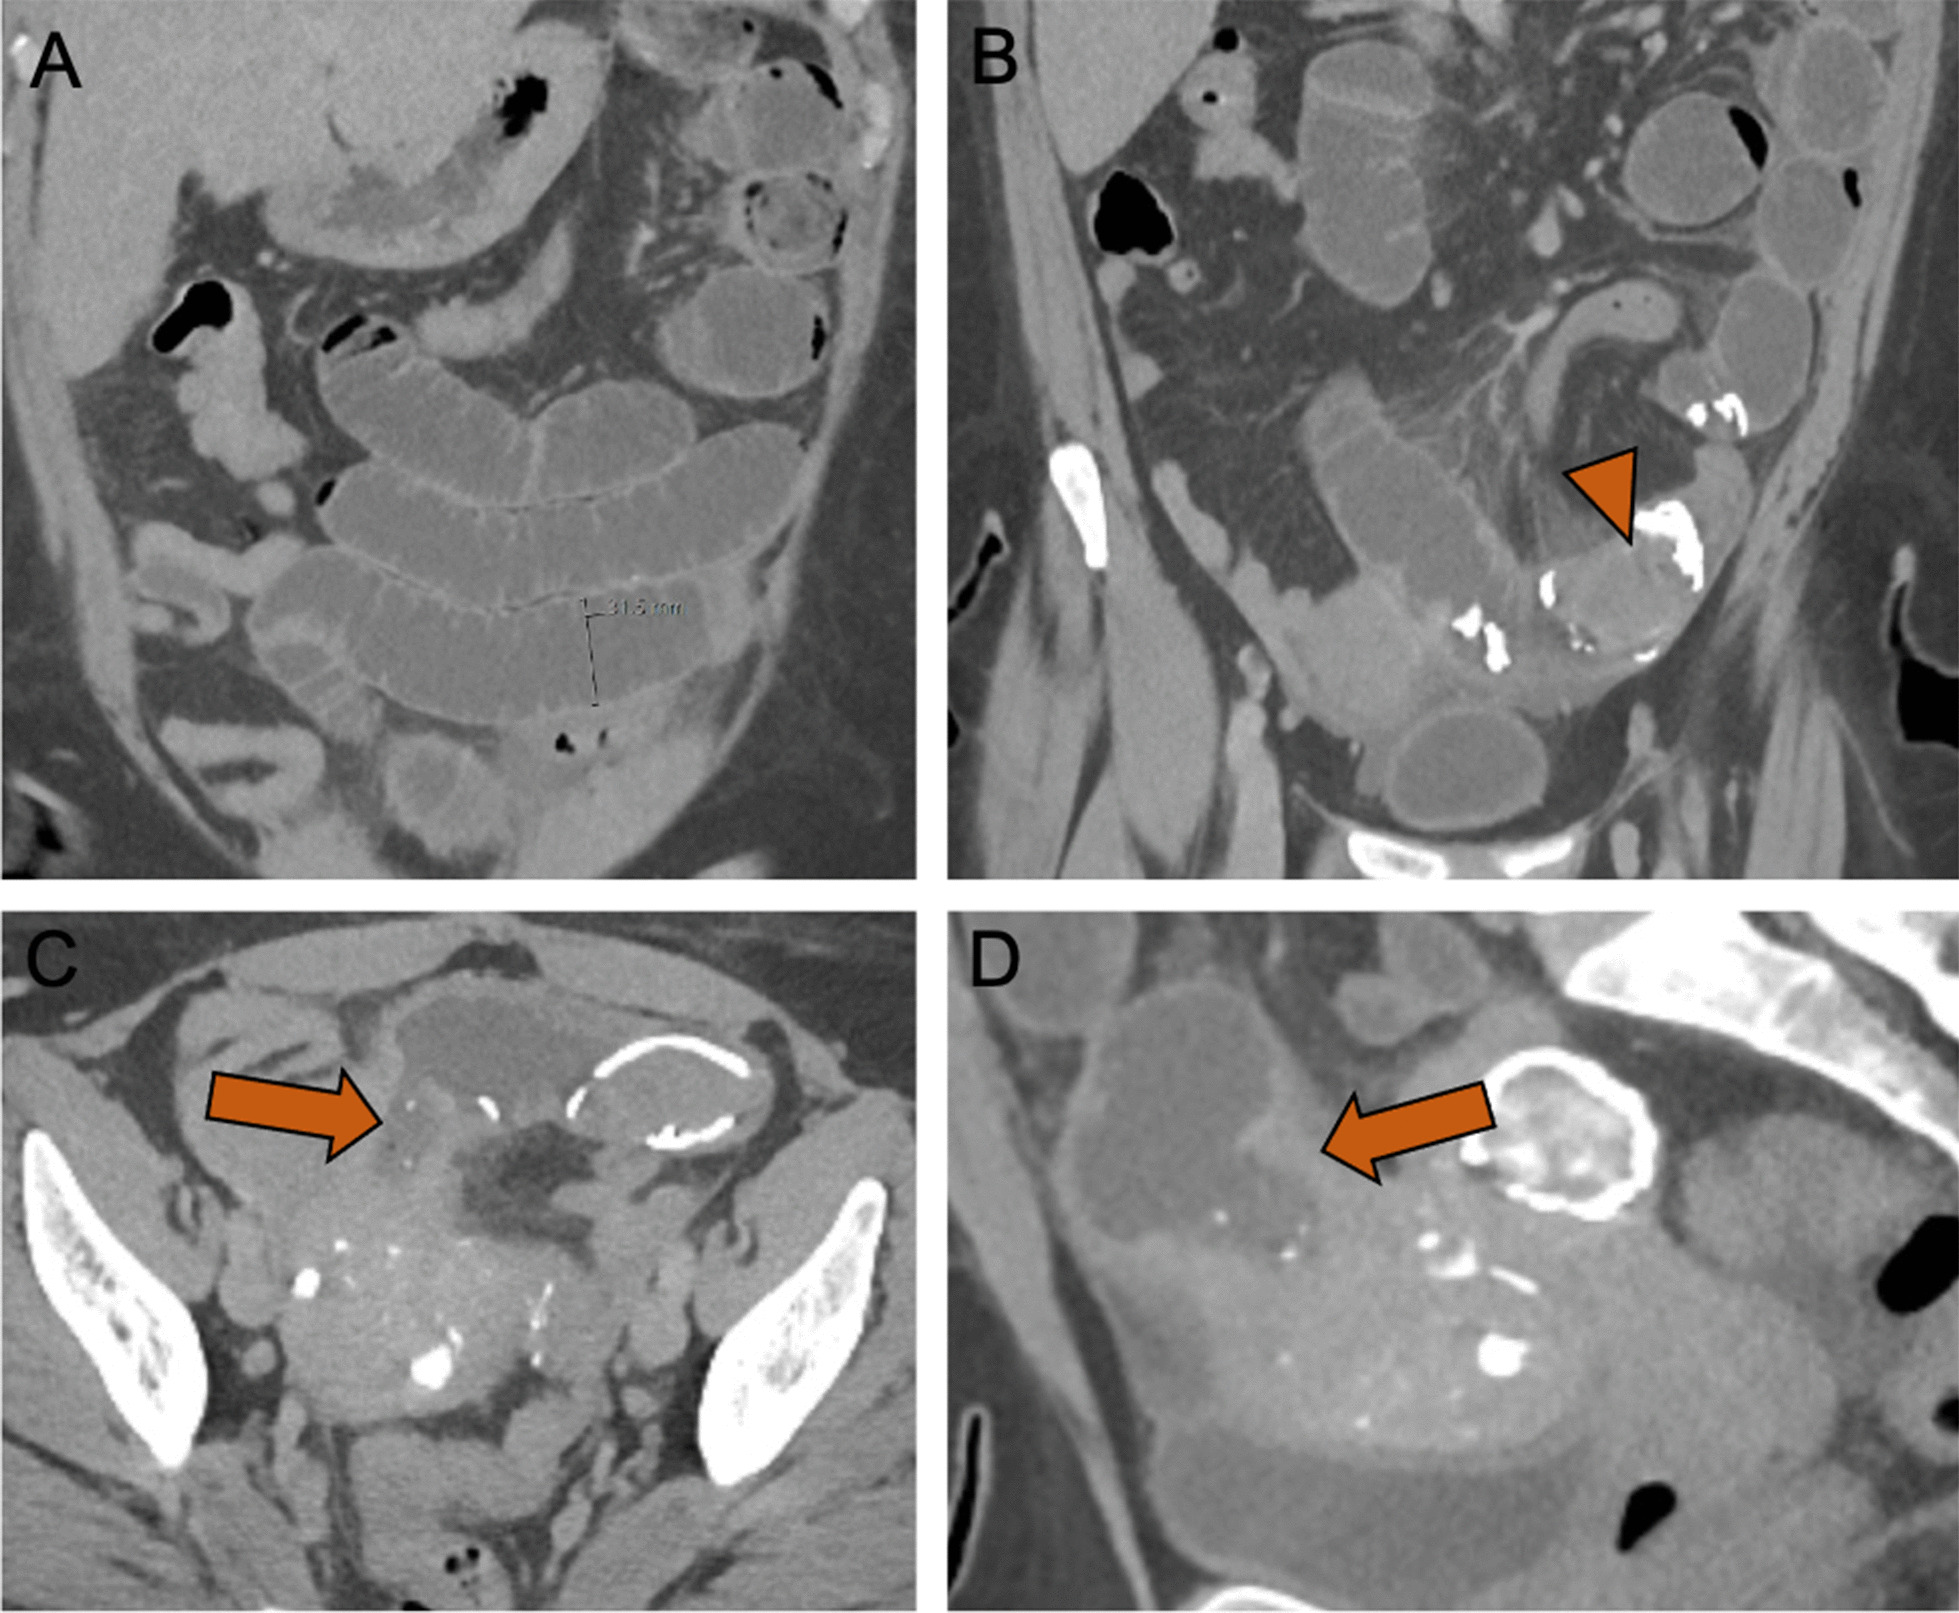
Contrast-enhanced CT images with coronal (A, B), axial (C), and sagittal (D) images. A Small bowel obstruction with dilated small bowel loop measuring up to 3.2 cm. B Small bowel obstruction secondary to an obstructing uterine leiomyoma in the ileal lumen (arrowhead). C, D Migration of leiomyoma into the ileal lumen due to an uteroenteric fistula between the uterine fundus and the ileum (arrows)
